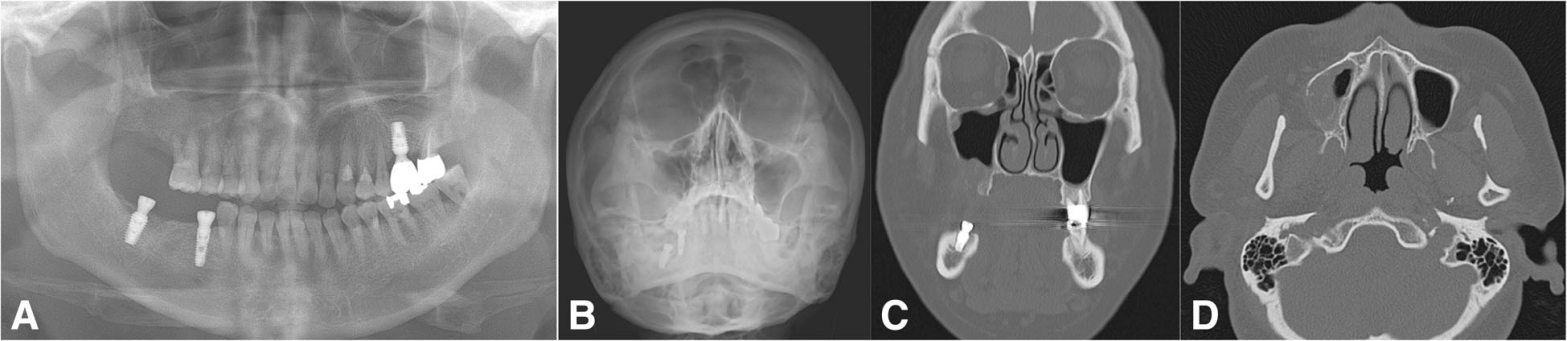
Chronic oronasal fistula after second molar extraction with several points of alveolar bony resorption indicates odontogenic maxillary sinusitis. Preoperative panoramic (a), Water’s (b), coronal CT scan (c), and axial CT scan (d) views
